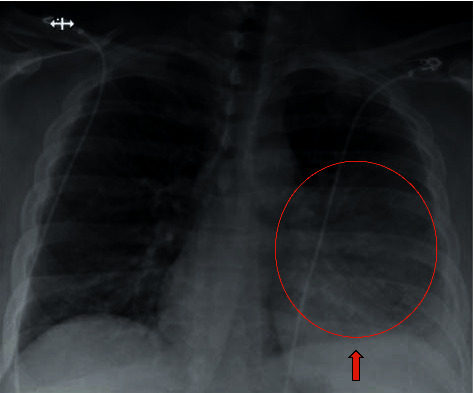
Chest X-ray 2 views revealing left lower lobe pneumonia with trace left pleural effusion with no pneumothorax or osseous abnormalities.
